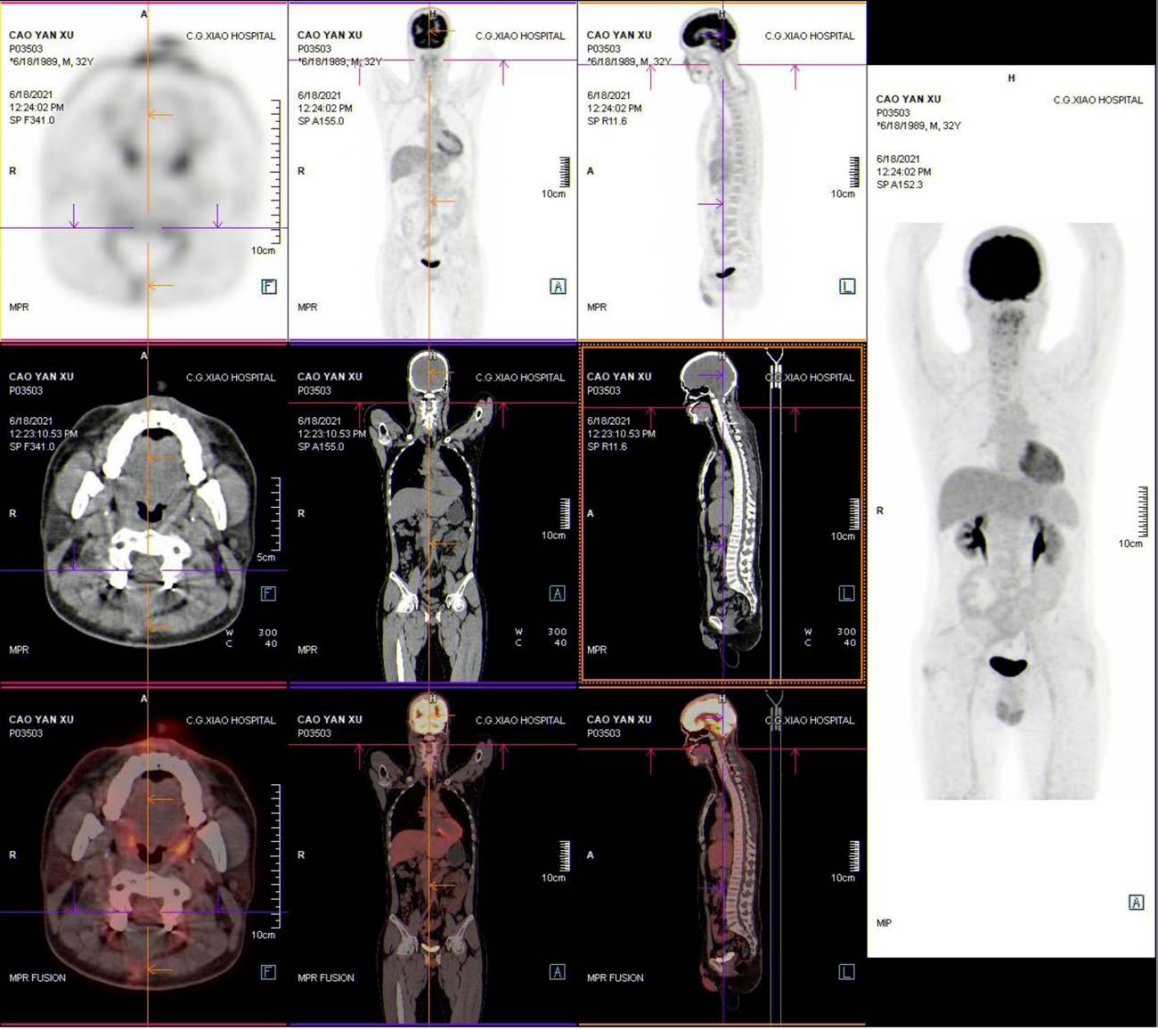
Postoperative cervical positron emission tomography–computed tomography 2 wk after surgery. The lesion did not involve other organs, and complete intraspinal surgical resection was performed.
